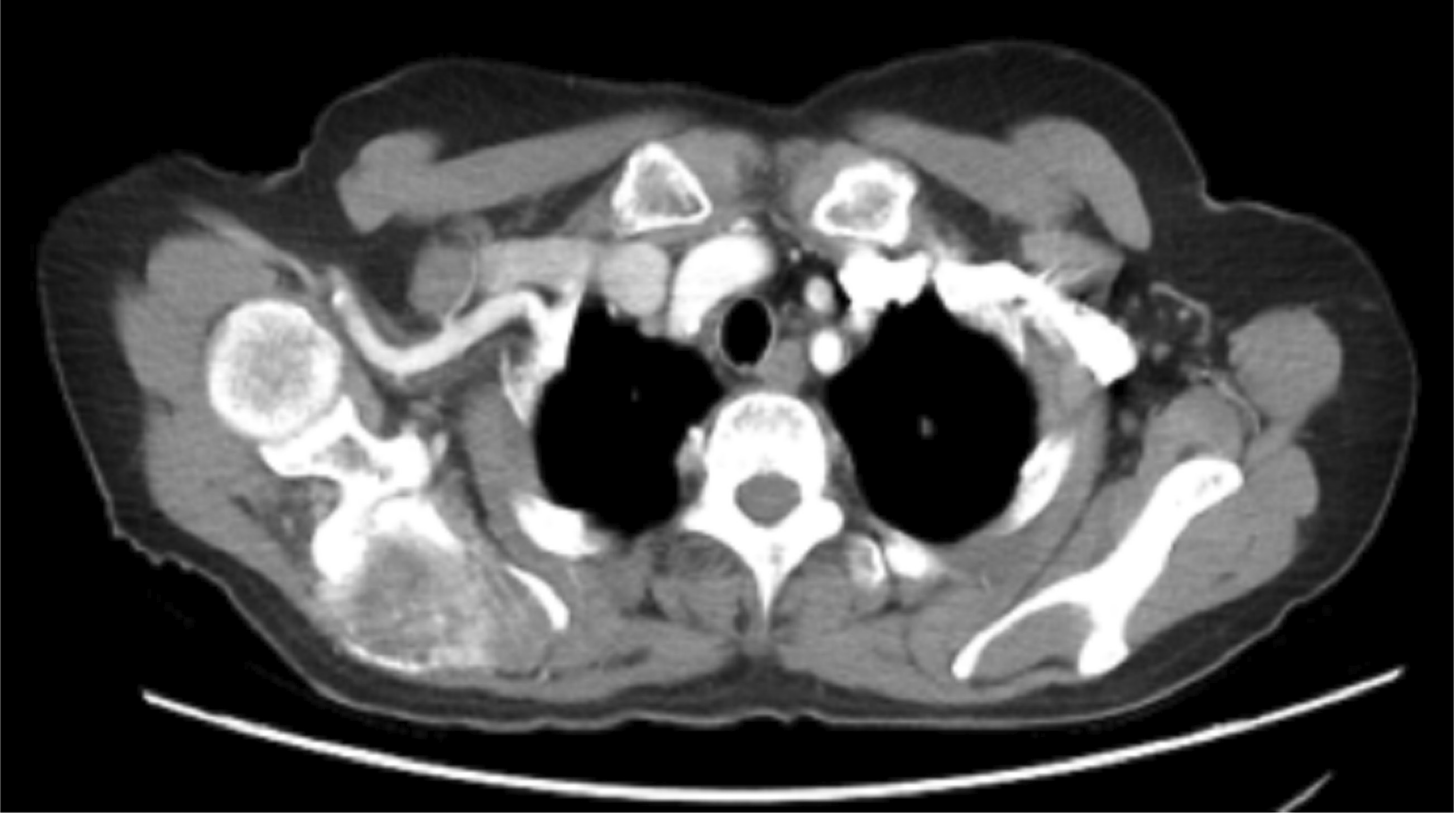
CT image of the chest in a 68‐year‐old female, it revealed osseous destruction and soft tissue mass in the right scapula, suggestive of bone tumor.
